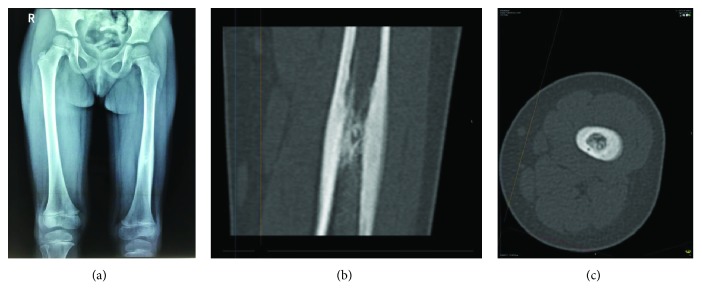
(a–c) Osteoid osteoma of the diaphysis of the left femur. X-ray with cortical thickening and CT with the nidus, surrounded by thickened cortices.
